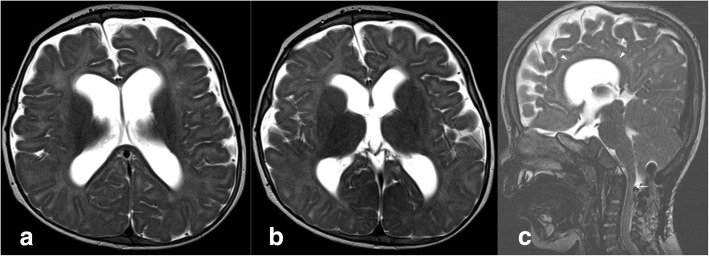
Brain MRI of an MPS VII patient at 12 months acquired post-surgery. On MR T2-weighted images acquired on the axial plane (a, b) through lateral ventricles and basal ganglia, enlargement of subarachnoid spaces and dilatation of the ventricular system are visible. On the sagittal plane (c) the corpus callosum is thinned and dysmorphic (arrowheads), and at the C1–C2 level, a cervical canal stenosis is still detectable (arrow)
